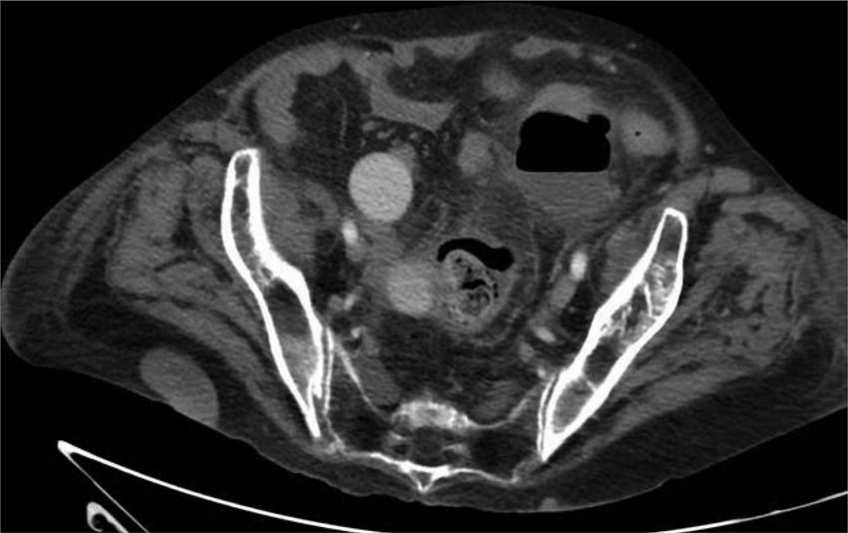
CT with abscess developed after initial antibiotic treatment.
